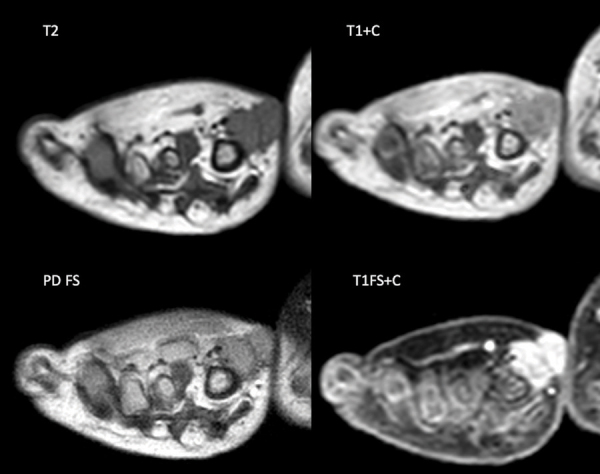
Coronal MRI images of the lesion showing isointense aspect on T2 (top left) and PD FS (down left) and bright enhancement on T1 (right).
